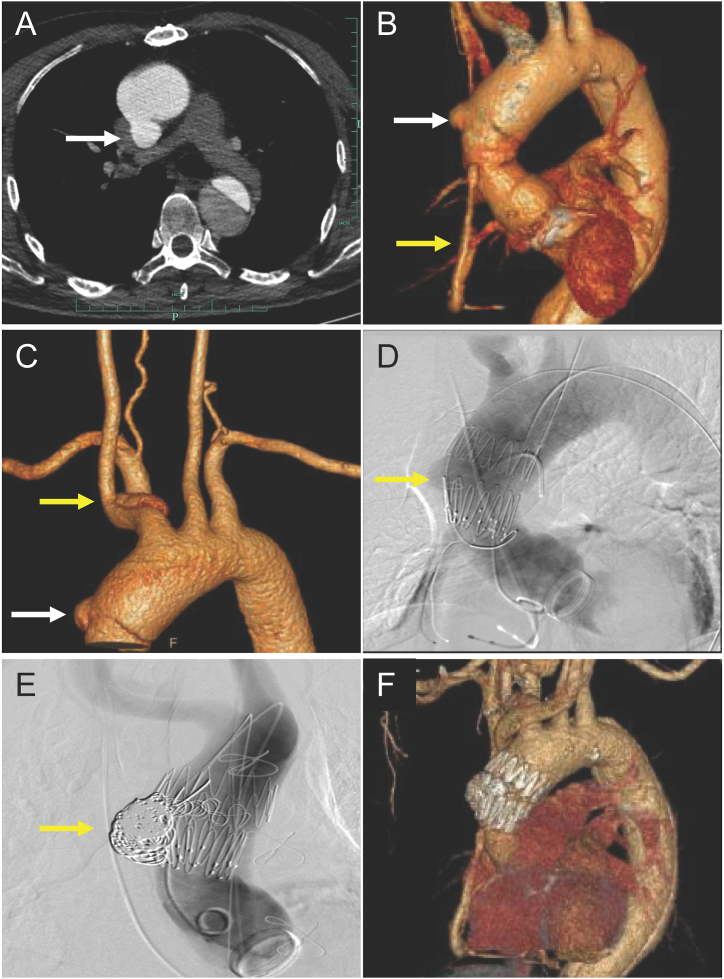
Images showing the anastomosis pseudoaneurysm and the stent graft. A. Computed tomography angiography showing the transverse view of the pseudoaneurysm (white arrow). B. Three-dimensional reconstruction showing the pseudoaneurysm (white arrow) and the coronary artery bypass graft (yellow arrow). C. Three-dimensional reconstruction showing the pseudoaneurysm (white arrow) and a residual false lumen in the brachiocephalic artery (yellow arrow). D. Angiography image showing the stent graft location and the endoleak (yellow arrow). E. Angiography showing the interlock coils (yellow arrow) packed into the pseudoaneurysm. F. Follow-up computed tomography reconstruction showing the stent graft in situ. (For interpretation of the references to colour in this figure legend, the reader is referred to the Web version of this article.)
